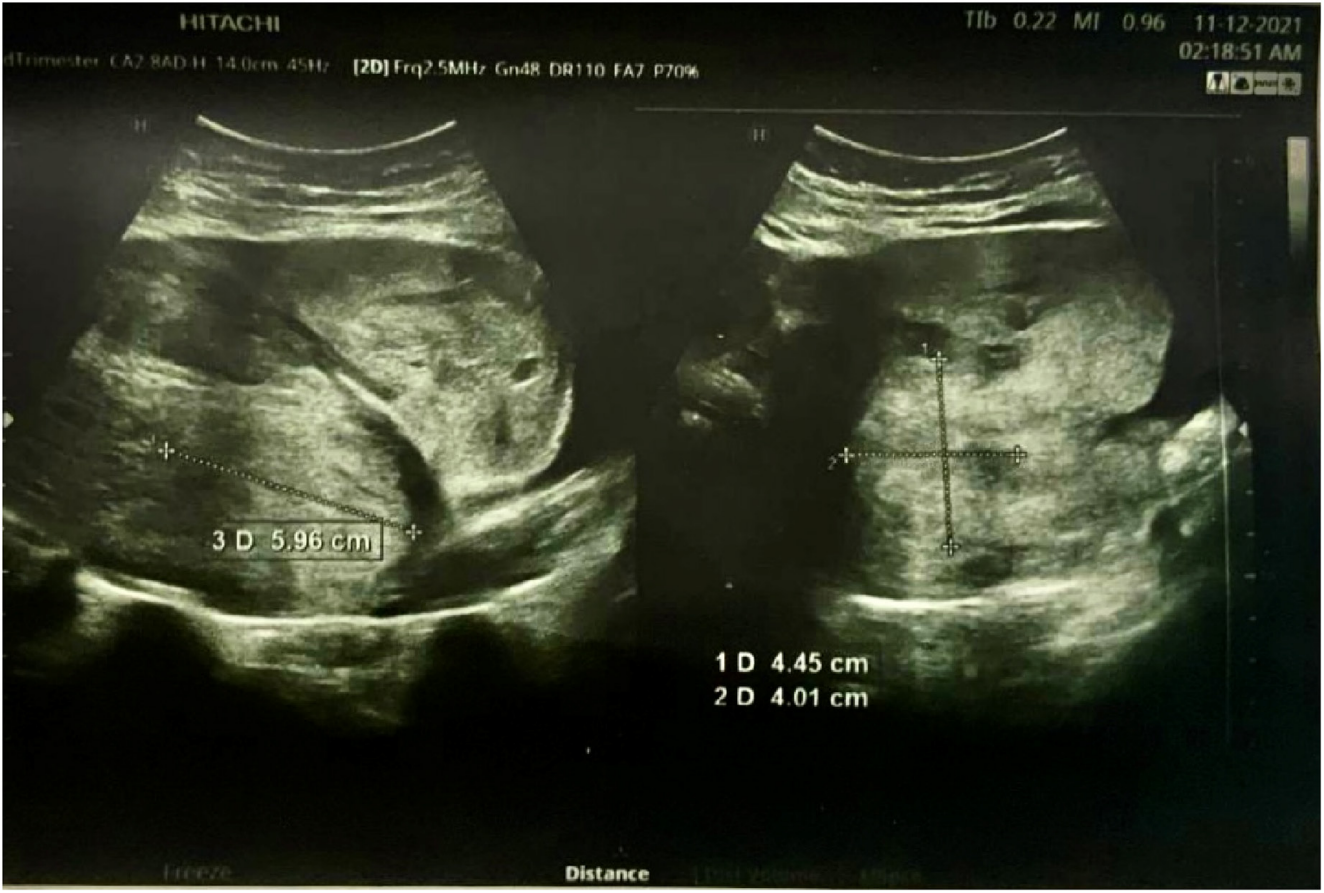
Ultrasound scan of the uterus showing a large blood clot seen at the anterior lower uterine segment between the anterior wall of the uterus and the urinary bladder.
